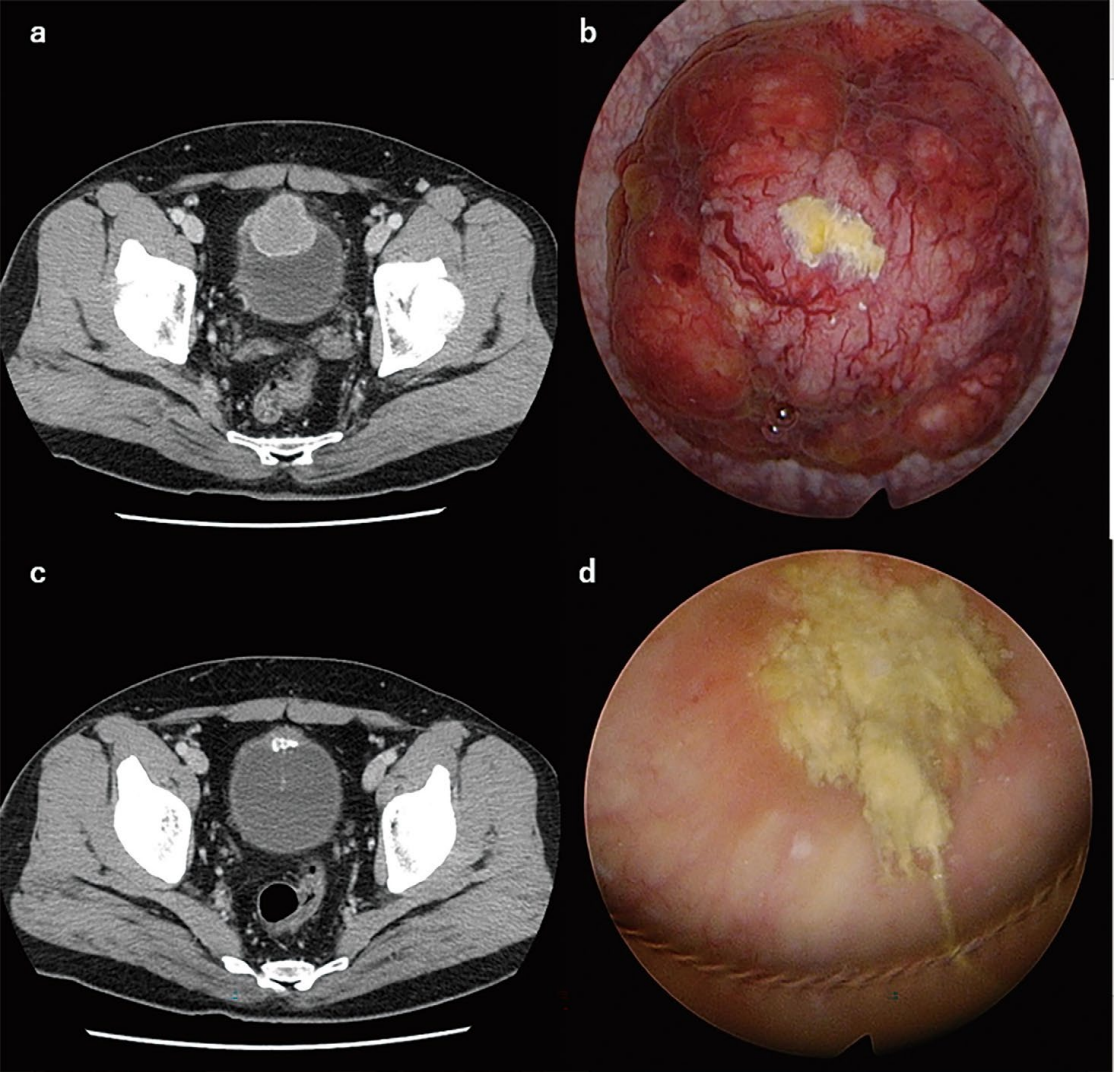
Pelvic computed tomography and cystoscopy images showing the bladder tumor (a, b) before transurethral resection and (c, d) after chemotherapy.
